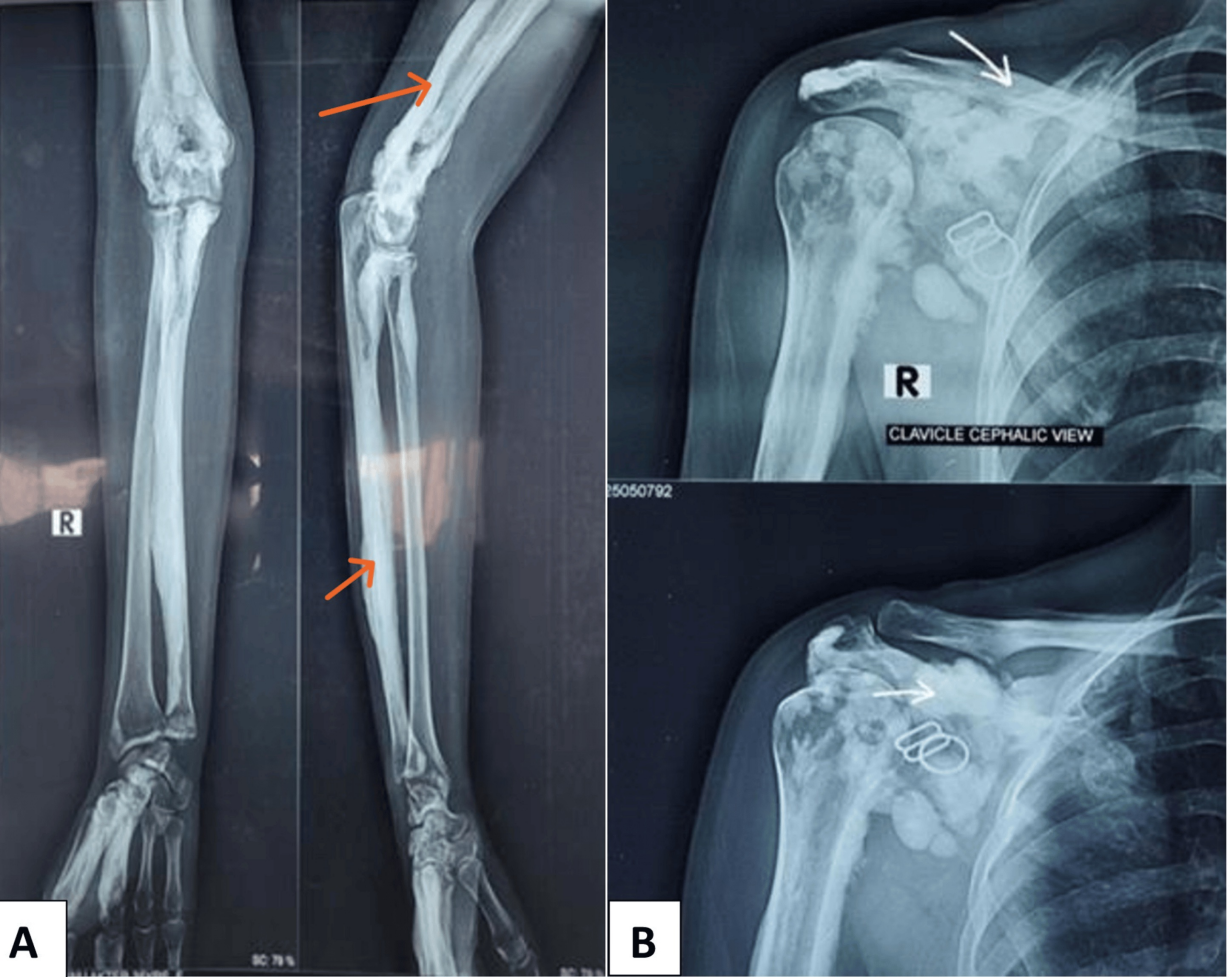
The X-rays show hyperostosis on the bones that looks like dripping candle wax, extending from the ulna to the lower humerus, and affecting the capitate, 2nd and 3rd metacarpal bones in Image A (orange arrows). Image B shows similar changes along the right clavicle, scapula, and humerus (white arrows).
